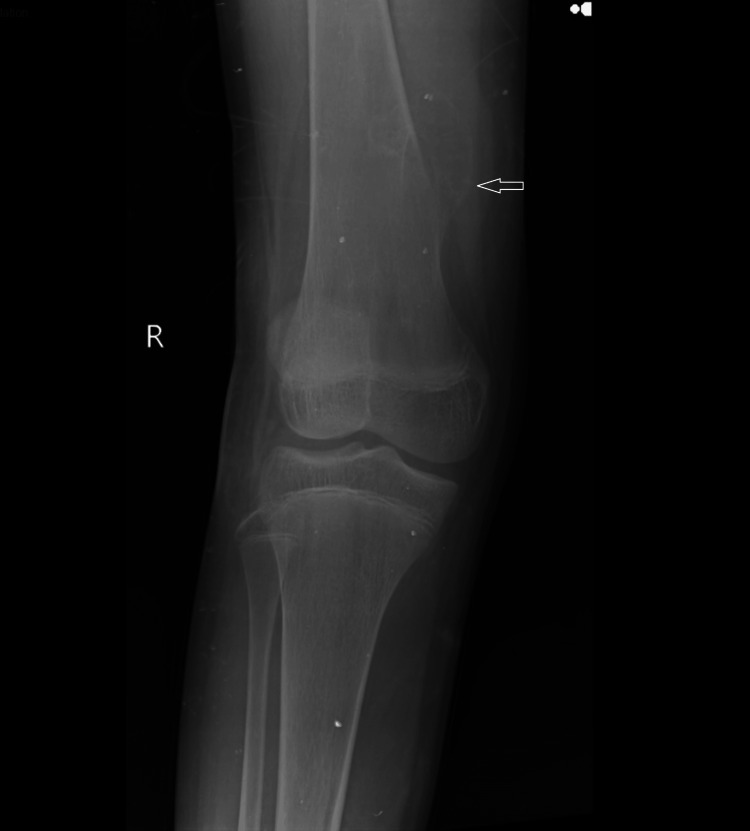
The AP view radiograph of the right knee with the distal femur and the proximal tibia in an immature skeleton shows a sessile bony outgrowth in the meta-diaphyseal location of the distal femur on the medial side. AP: anteroposterior
